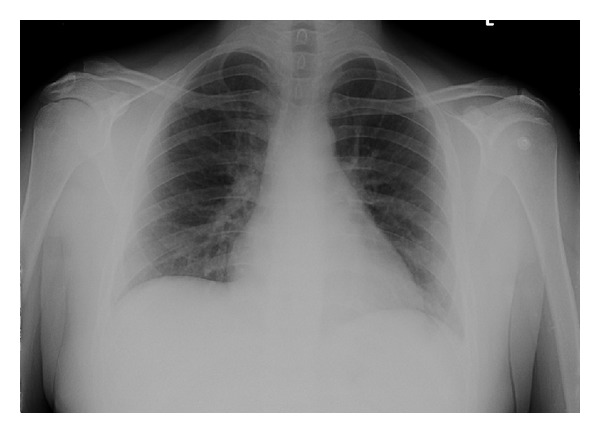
Patient's Chest radiograph on admission with no infiltrates.
